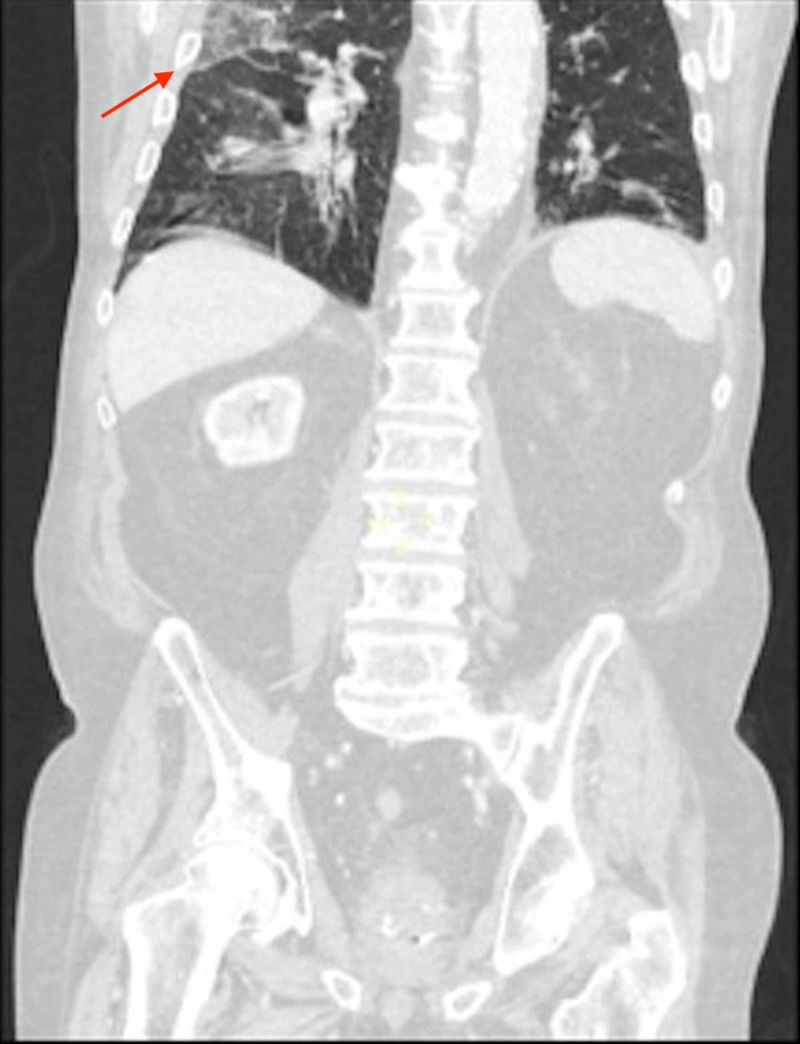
CT - Coronal View Peripheral ground-glass opacification within the right upper and lower lobes (red arrow) on coronal view. Combined with the findings on the axial view, these appearances were considered equivocal for COVID-19 infection.
